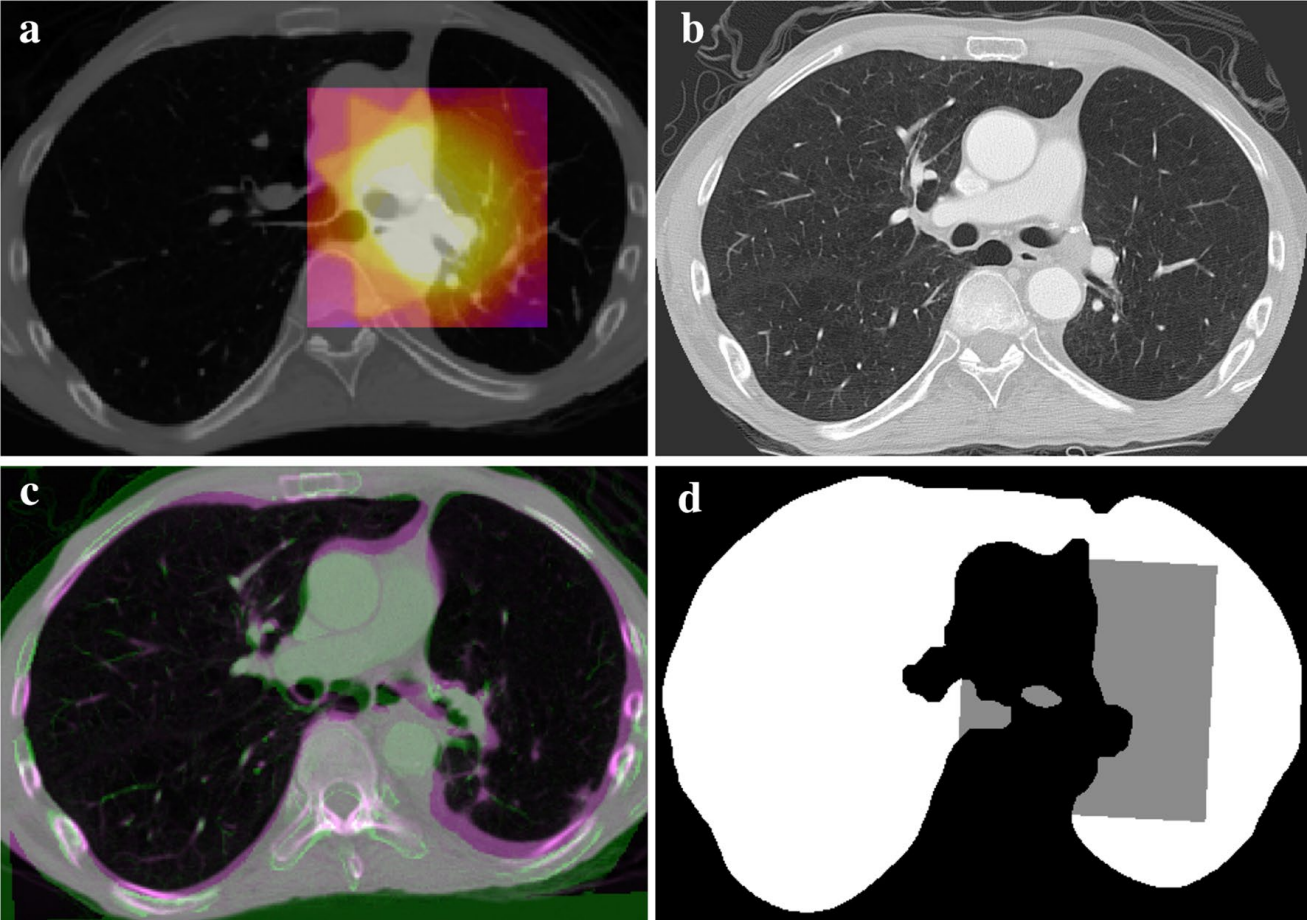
Lung Segmentation and registration results: (a) shows for a representative patient a treatment planning chest CT slice through a tumor located within the hilar region. The colorized overlay is the planned dose distribution over a 10 × 10 × 10 cm^3^ region centered on the isocenter. (b) An axial CT slice at approximately the same anatomical location at the 8-month follow-up time point and in the same patient as (a). (c) Depicts the result of the rigid body registration of the 8-month follow-up CT to the panning CT at the same anatomical slice location. (d) The lung volume mask in white and gray for the 8-month follow-up image. The computed dose grid is transformed from the planning CT image space to the follow-up CT image space and the gray pixels show where the dose grid aligns to pixels in the lung mask of the follow-up image. The region of gray pixels is where the dose–response data is computed.
